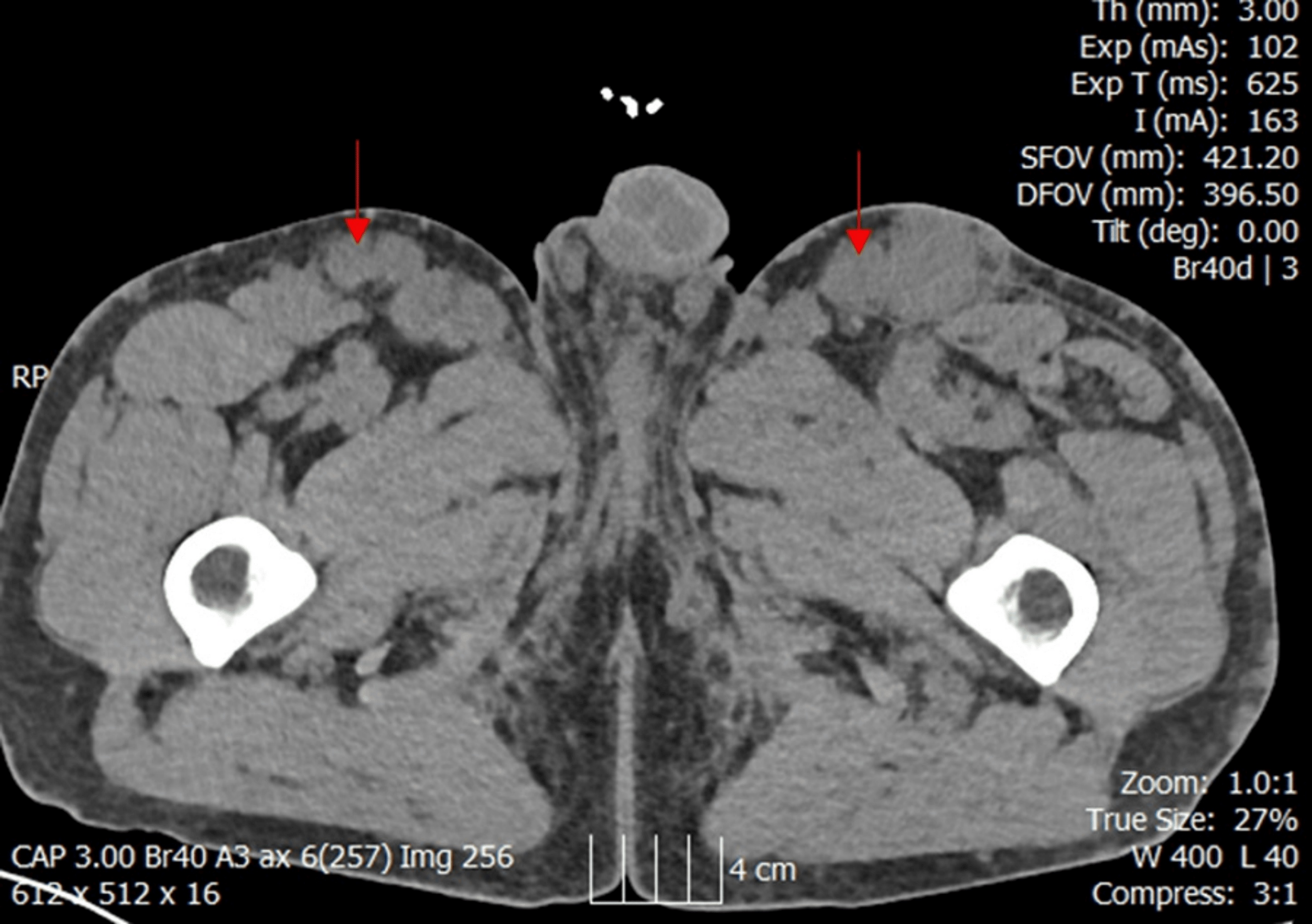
Axial CT scan showing inguinal lymphadenopathy
